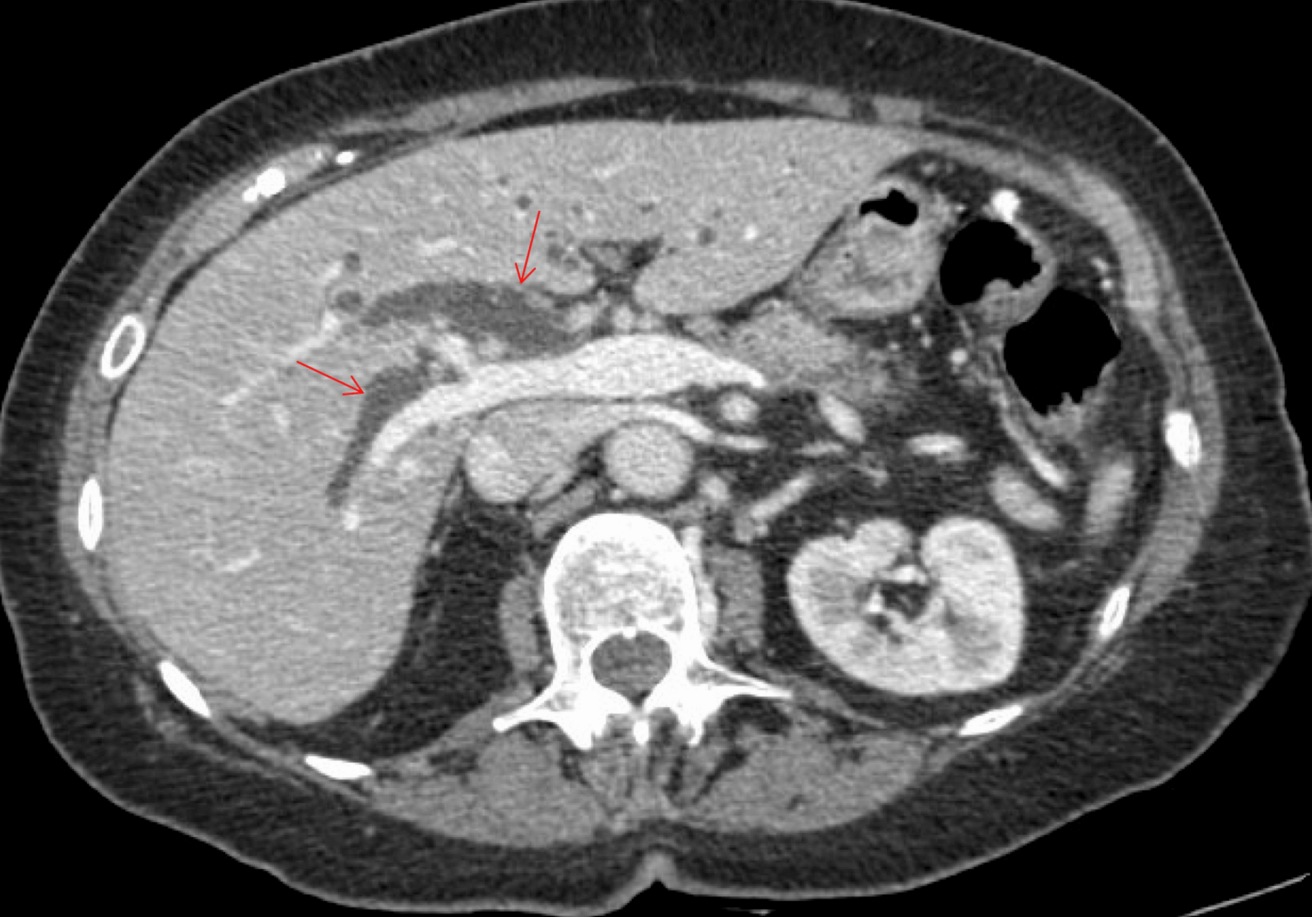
CT abdomen and pelvis, axial view in venous phase. The red arrows point to significant intrahepatic and common bile duct dilation.
